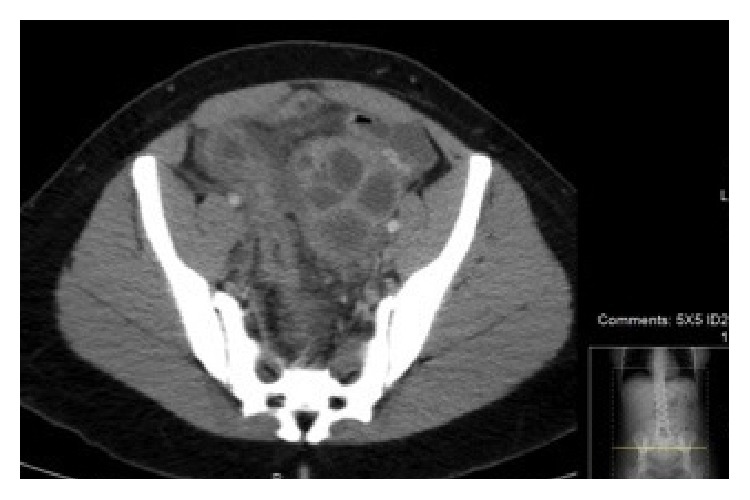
CT scan showing a large multicystic left ovarian mass measuring 6 × 9 cm.
